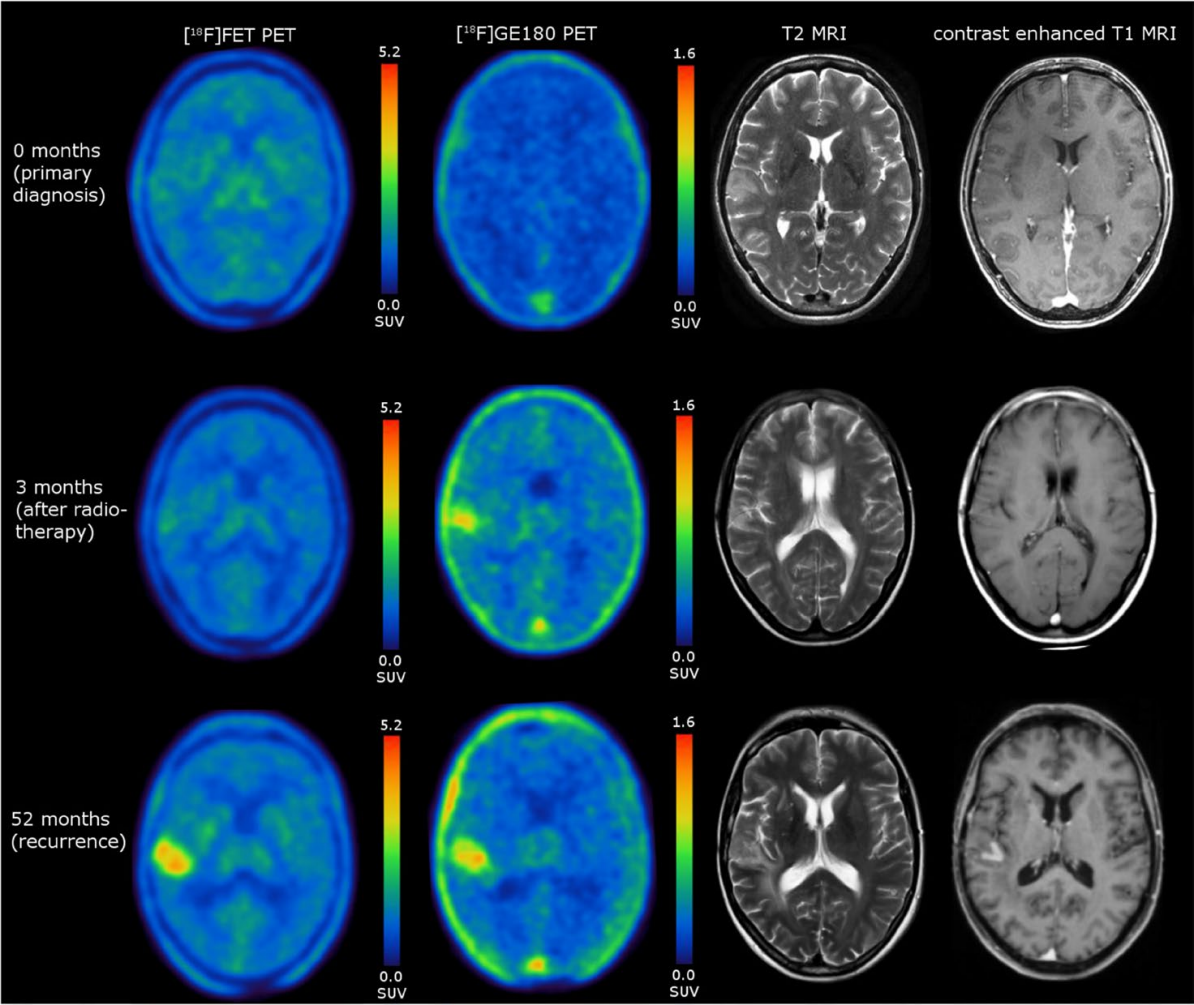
A 51-year-old female patient presented with newly onset seizure. MR imaging revealed a T2-hyperintense lesion without contrast enhancement in the right temporoinsular region showing low uptake on both amino acid PET with [^18^F]FET and TSPO PET with [^18^F]GE180. Stereotactic biopsy revealed an IDH-wildtype glioma, *MGMT*-methylated, with a TERT promoter mutation and a proliferation index of 10%, consistent with a WHO grade 4 glioblastoma according to the current classification [1]. After radiochemotherapy according to EORTC/NCIC protocol [2], [^18^F]FET uptake remained continually low, while [^18^F]GE180 PET showed a focally increased uptake in the treated area. The patient remained stable for a remarkably long period, until tumor recurred locally after 4.3 years. At recurrence, both [^18^F]FET PET and [^18^F]GE180 PET showed an equally strong uptake.
